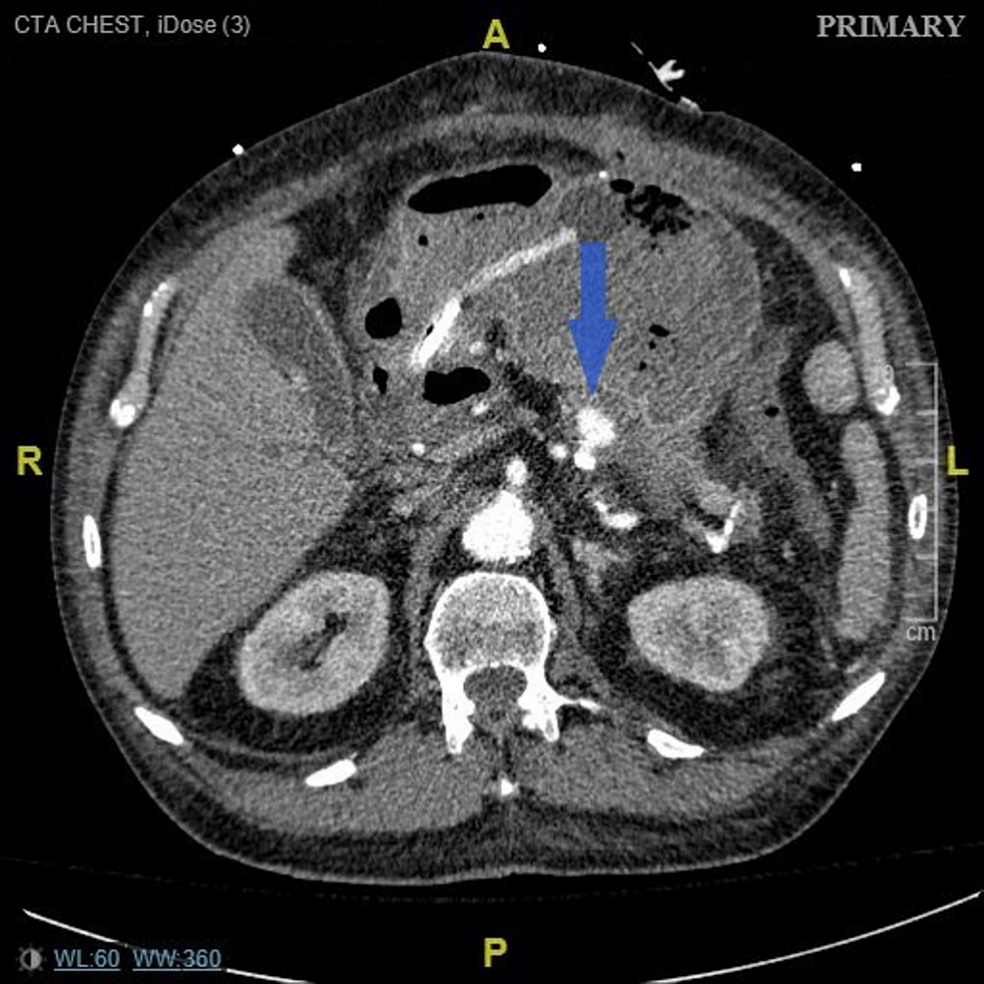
Computed tomography angiography showing splenic artery pseudoaneurysm measuring 3.6 x 1.8 x 1.4 cm (blue arrow)
